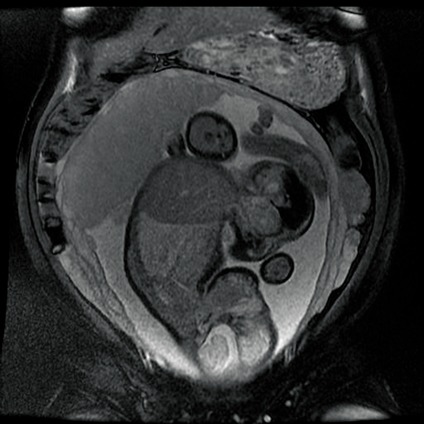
Fetal MRI–gestational age 21 weeks, viable fetus and parasite fetus (longitudinal plan)
